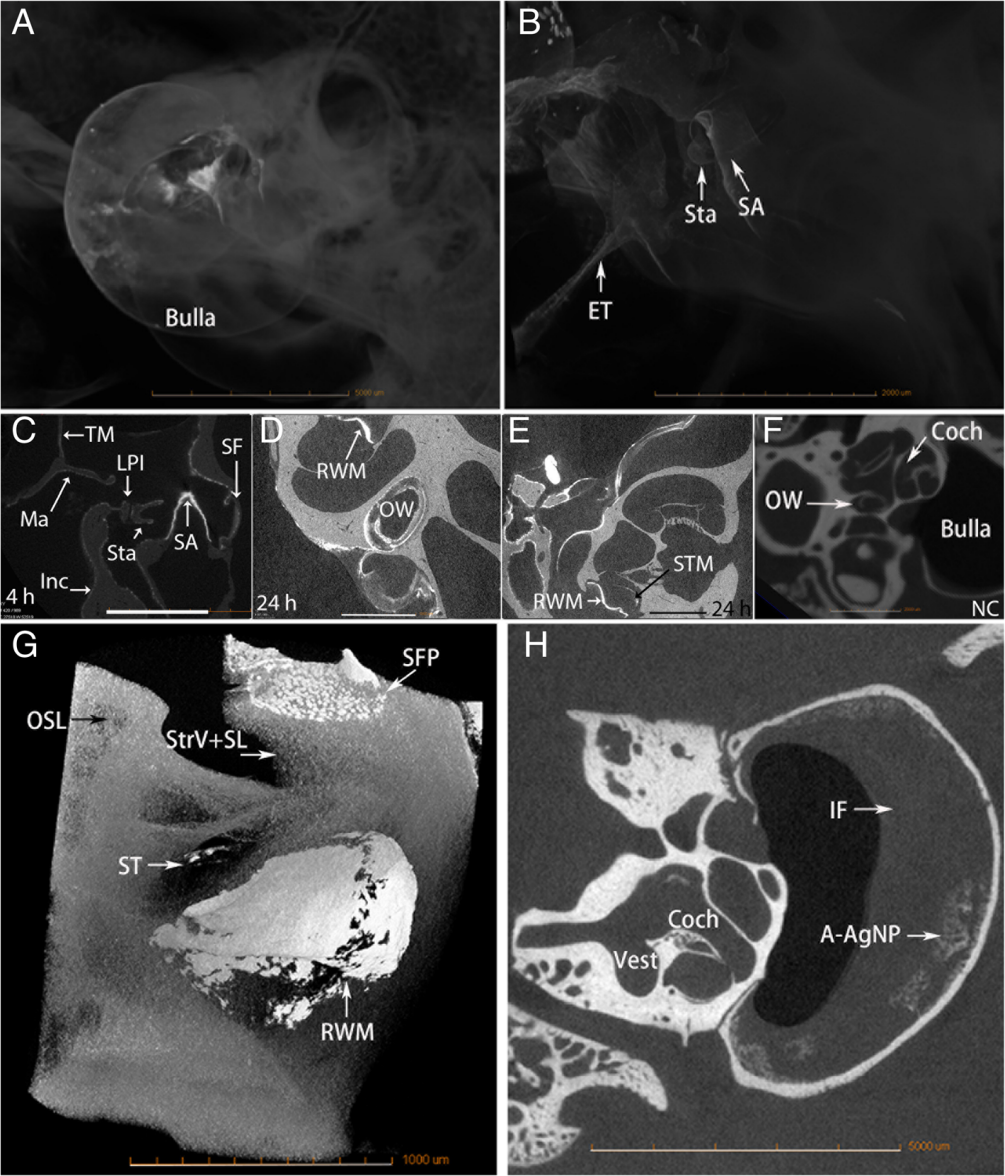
Distribution of Ag NPs in the ear after transtympanic injection shown by micro CT. Either 370.7 mM (A-E, G) or 37.1 mM (H) of Ag NPs were injected at a volume of 50 μl. At 4 h post-administration (370.7 mM), AgNPs generated bright signal that appeared in the bulla, tympanic membrane (TM) Eustachian tube (ET), and the ossicular chain including malleus (Ma), incus (Inc) and stapes (Sta) (A-C). Abundant Ag NPs were found in the stapedial artery (SA) (C). At 24 h (370.7 mM), abundant distribution of AgNPs was detected in the round window membrane (RWM), oval window (OW), and scala tympani medial wall (STM) of the cochlea (D, E, G). On 7 d (37 mM), middle ear infiltration (IF) and AgNP aggregation (A-AgNPs) were observed (H). No Ag NPs were detected in the ear of non-treatment control (NC) (F). Coch: cochlea; LPI: lenticular process of incus; SF: stapes footplate; ST: scala tympani. Scale bars = 5 mm (A), 2 mm (B, F), 1 mm (C-E). A-F, H: 4x, Pixel size 21.8498; G: 10x, pixel size 1.7 um.
